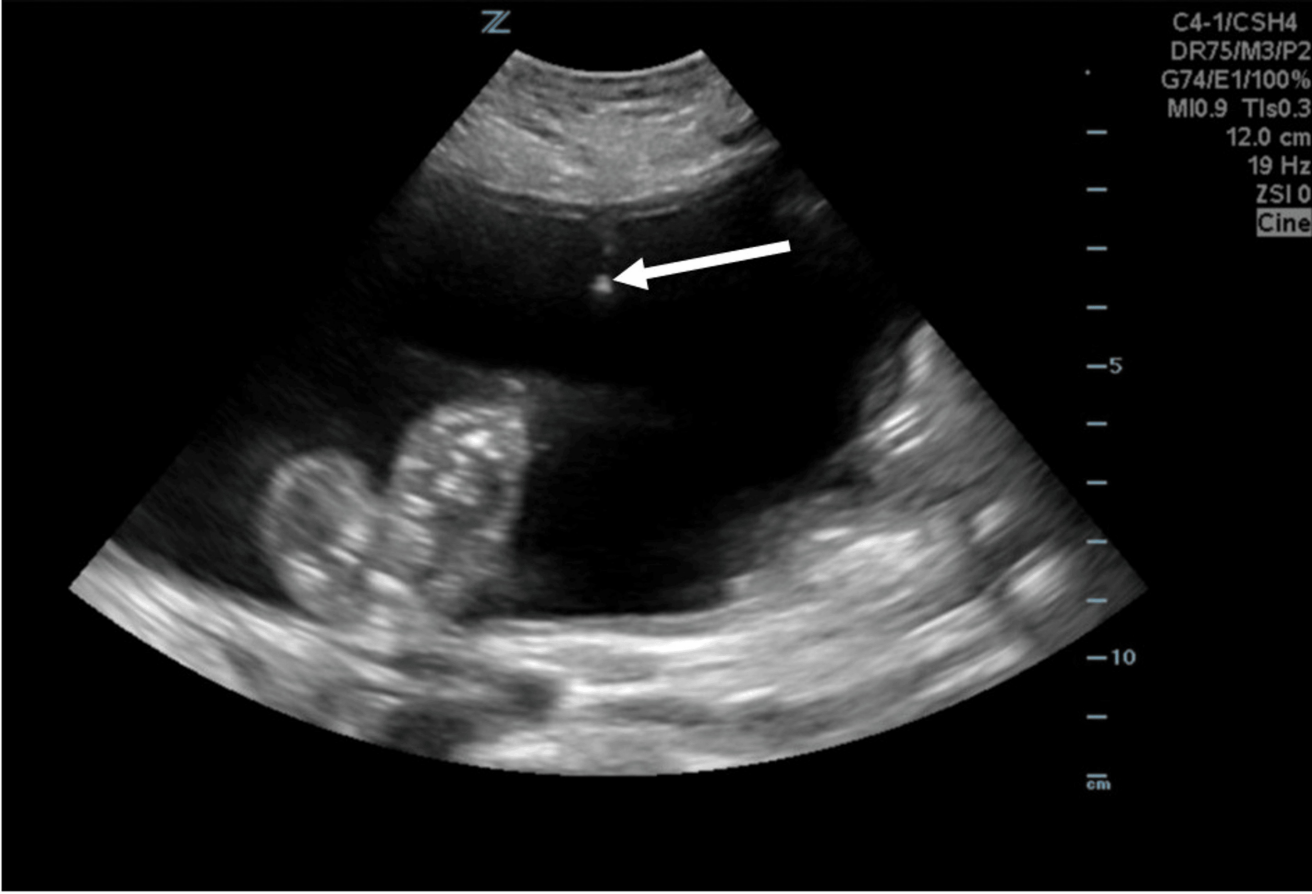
Ultrasound-guided paracentesis Ultrasound-guided paracentesis with the needle tip (white arrow) of the paracentesis needle
